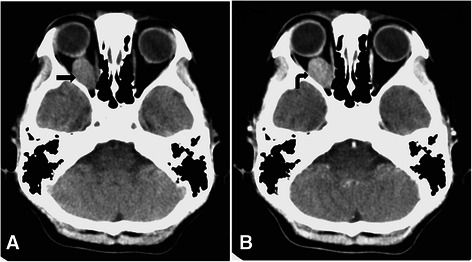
Intraorbital granular cell tumor on brain computed tomography. a Pre-contrast and b post-contrast axial brain computed tomography scans show a well-defined, ovoid, retrobulbar nodule (*arrow*) with slight contrast enhancement (*curved arrow*). The right globe is not indented by the tumor
